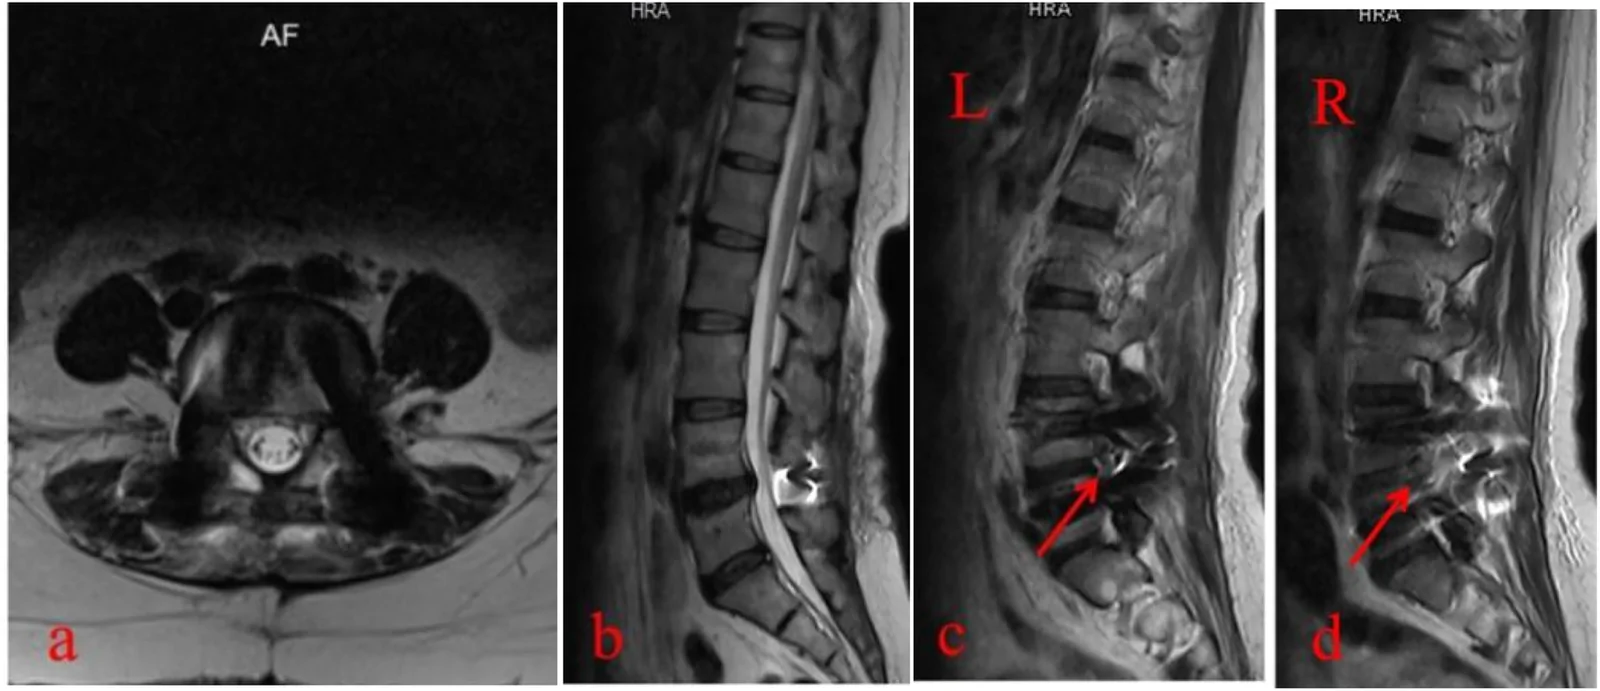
A postoperative MRI demonstrated adequate decompression of the central canal and ruled out epidural hematoma and residual disc–induced neural compression (a,b). The foraminal region showed blurred and irregular signal characteristics, which precluded accurate radiological interpretation (c,d).
